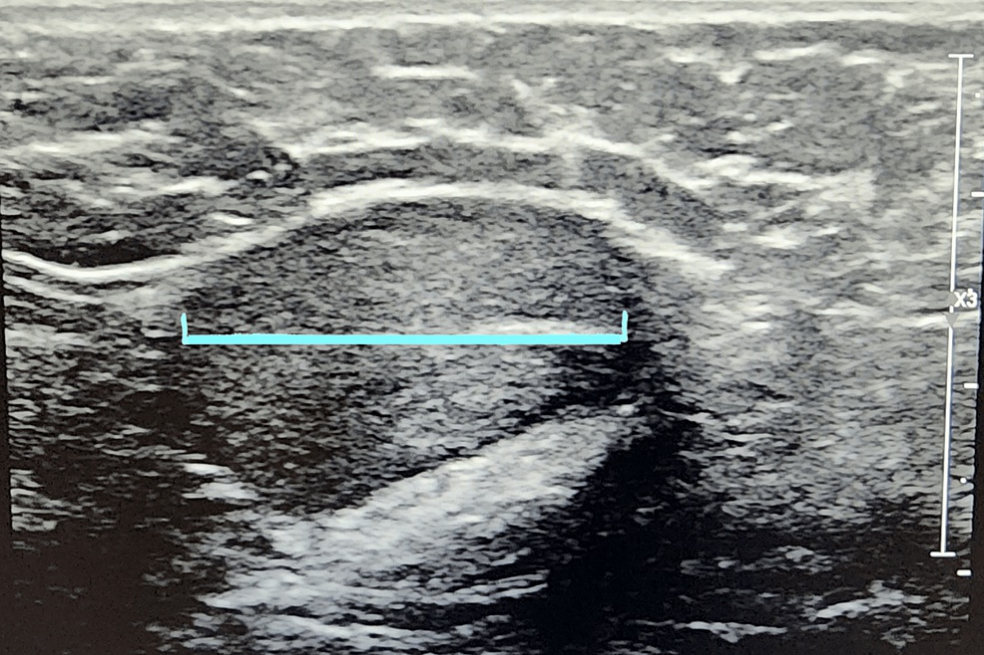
Right inguinal canal with right testicle ultrasound. Blue line showing the length of the right testicle.
